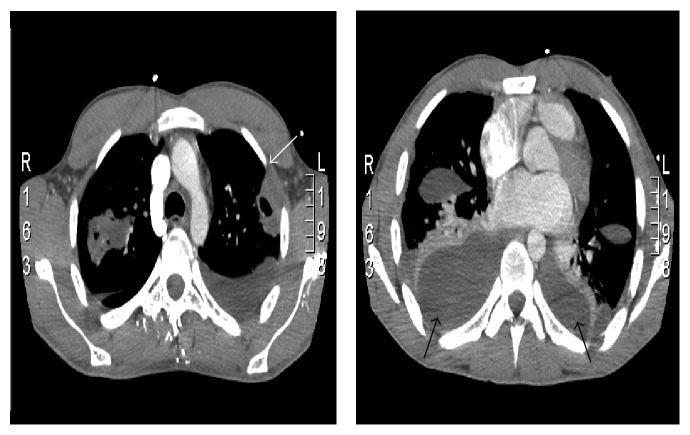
CT scan of the chest. Numerous bilateral pulmonary nodules (white arrow) can be seen with varying degrees of cavitation along with moderate bilateral pleural effusions and compressive atelectasis of both lung bases (black arrows).
